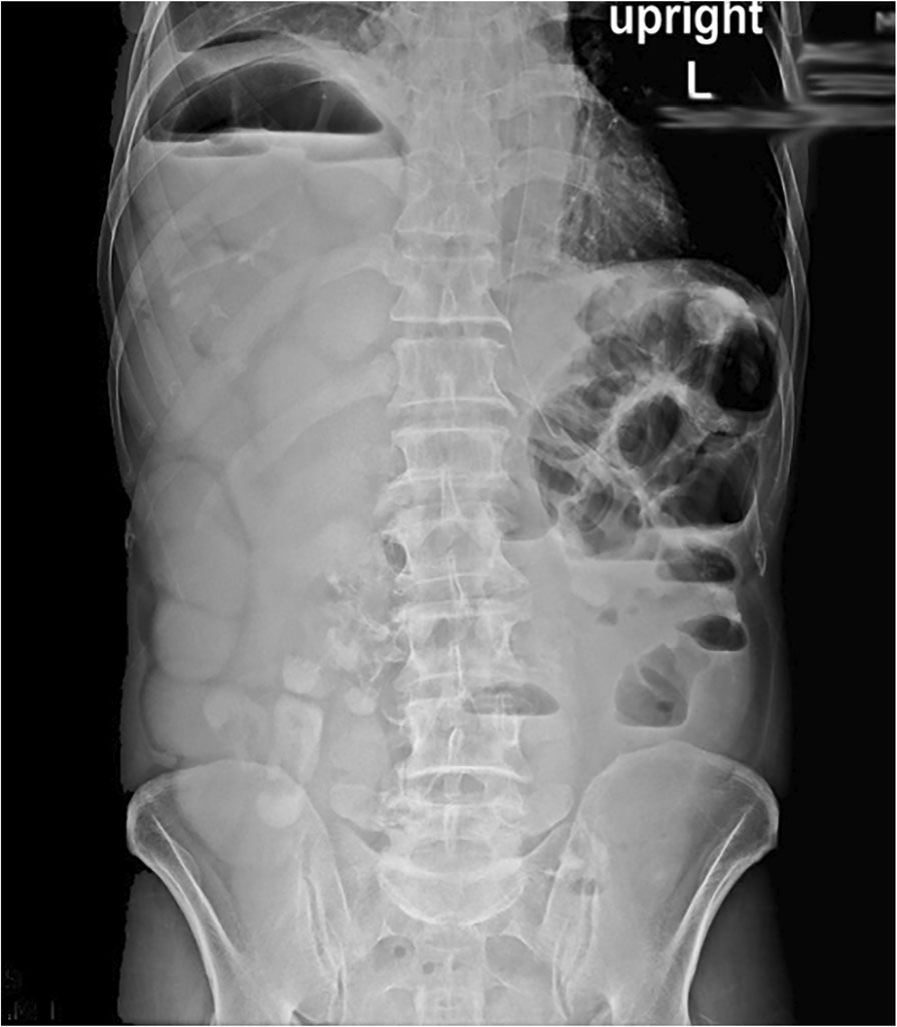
Abdominal X-ray: multiple air-fluid level and air above the liver on the right
